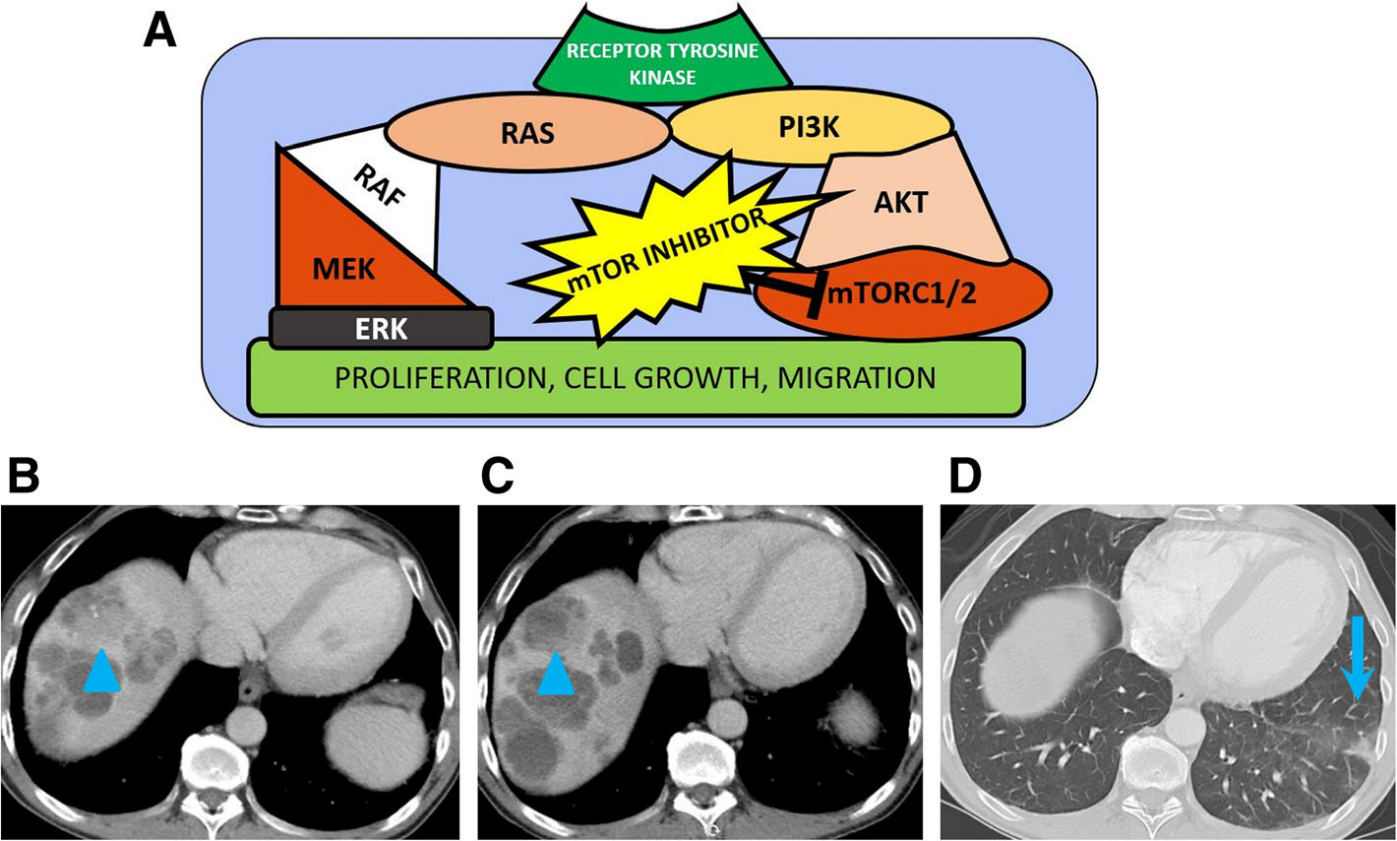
PI3K pathway. a Image showing the PI3K-ATK-mTORC pathway and the blockage of the effector mTORC1/2 by the mTOR inhibitors, such as everolimus. b–d Baseline and restaging contrast-enhanced CT images of a 60-year-old man with metastatic neuroendocrine tumor on everolimus show decreased enhancing component of a liver metastasis (arrowheads), representing response to treatment. Patient developed drug induced pneumonitis while on treatment (arrow) (d)
